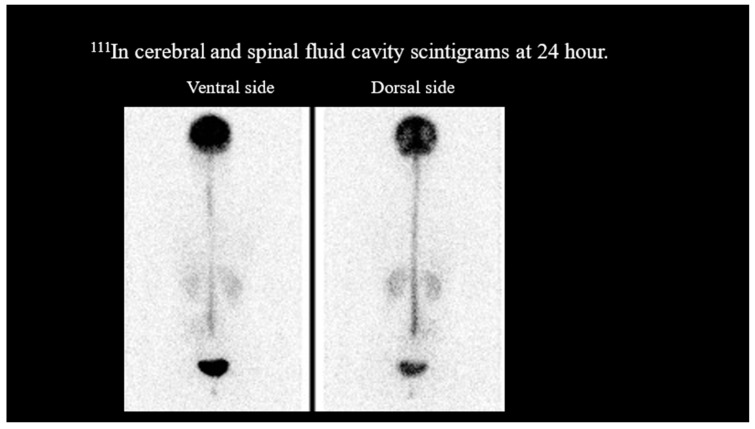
^111^In cerebrospinal fluid cavity scintigrams at 24 h. The residual radioisotope in the cerebrospinal fluid space was 15% at 24 h (normal, >30%), suggesting spinal fluid leakage.
